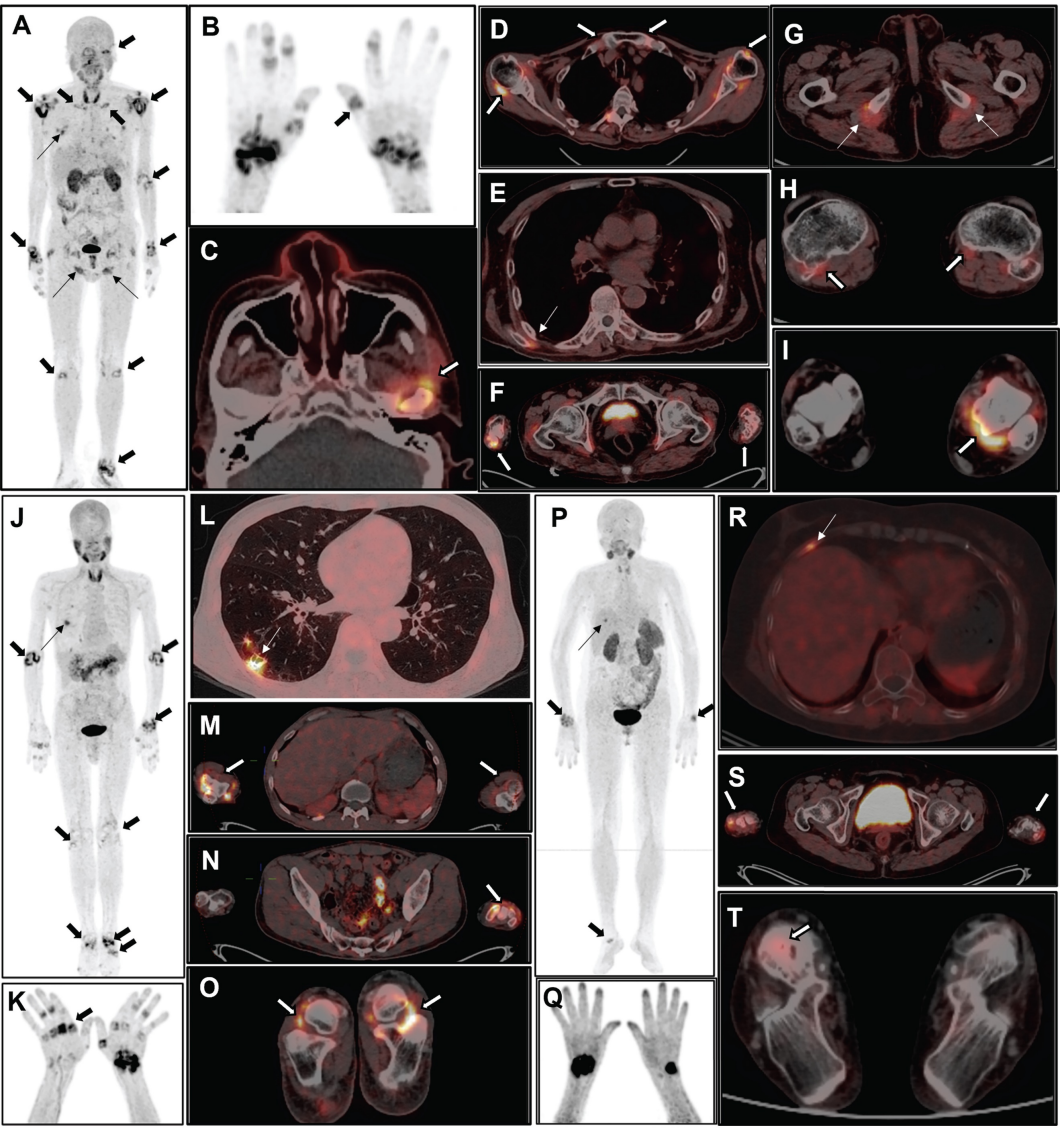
The maximum intensity projection (MIP) images (A and B) of a 60-year-old male participant (No. 7) reveal increased uptake in various joints (thick arrows), muscles and tissues (thin arrows). The participant has DAS28-ESR score of 5.6, DAS28-CRP score of 5.2, CDAI of 16, and SDAI of 219. Axial PET/CT fusion images demonstrate elevated FAPI-RGD uptake in the left temporomandibular joint (C), bilateral shoulder and sternoclavicular joints (D), bilateral paraspinal muscles of the subscapularis angle (E), bilateral carpal joints (F), bilateral tissues around the sciatic tuberosity (G), bilateral knee joints (H), and the left ankle joint (I). The MIP images (J and K) of a 53-year-old male participant (No. 14) reveal increased uptake in multiple joints (thick arrows) and right lung (thin arrow). The participant has DAS28-ESR score of 8.5, DAS28-CRP score of 7.2, CDAI of 69.5, and SDAI of 75.9. Axial PET/CT fusion images reveal increased FAPI-RGD uptake in the lower lobe of the right lung (L), bilateral elbow joints (M), left wrist joint (N), and bilateral ankle joints (O). The MIP images (P and Q) of a 54-year-old female participant (No. 5) show increased uptake in the joints (thick arrow) and right lower thorax (thin arrow). The participant has DAS28-ESR score of 3.3, DAS28-CRP score of 2.3, CDAI of 6.0, and SDAI of 6.5. Axial PET/CT fusion images reveal increased FAPI-RGD uptake in the region of the right rib fracture (R), bilateral wrist joints (S), and right ankle joint (T).
